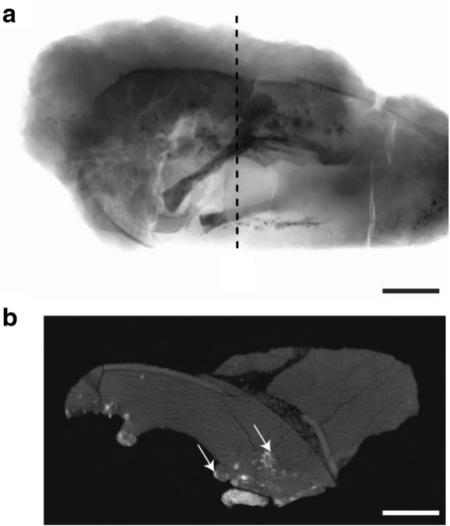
CT images of IVPP V20183. a, volumetric model generated from superimposed CT slices, with a black dashed line marking the position of the slice shown in B; scale bar = 5 cm; b, selected CT slice showing the limited density contrast between the bone and matrix (white arrows mark the bones); scale bar = 1 cm
